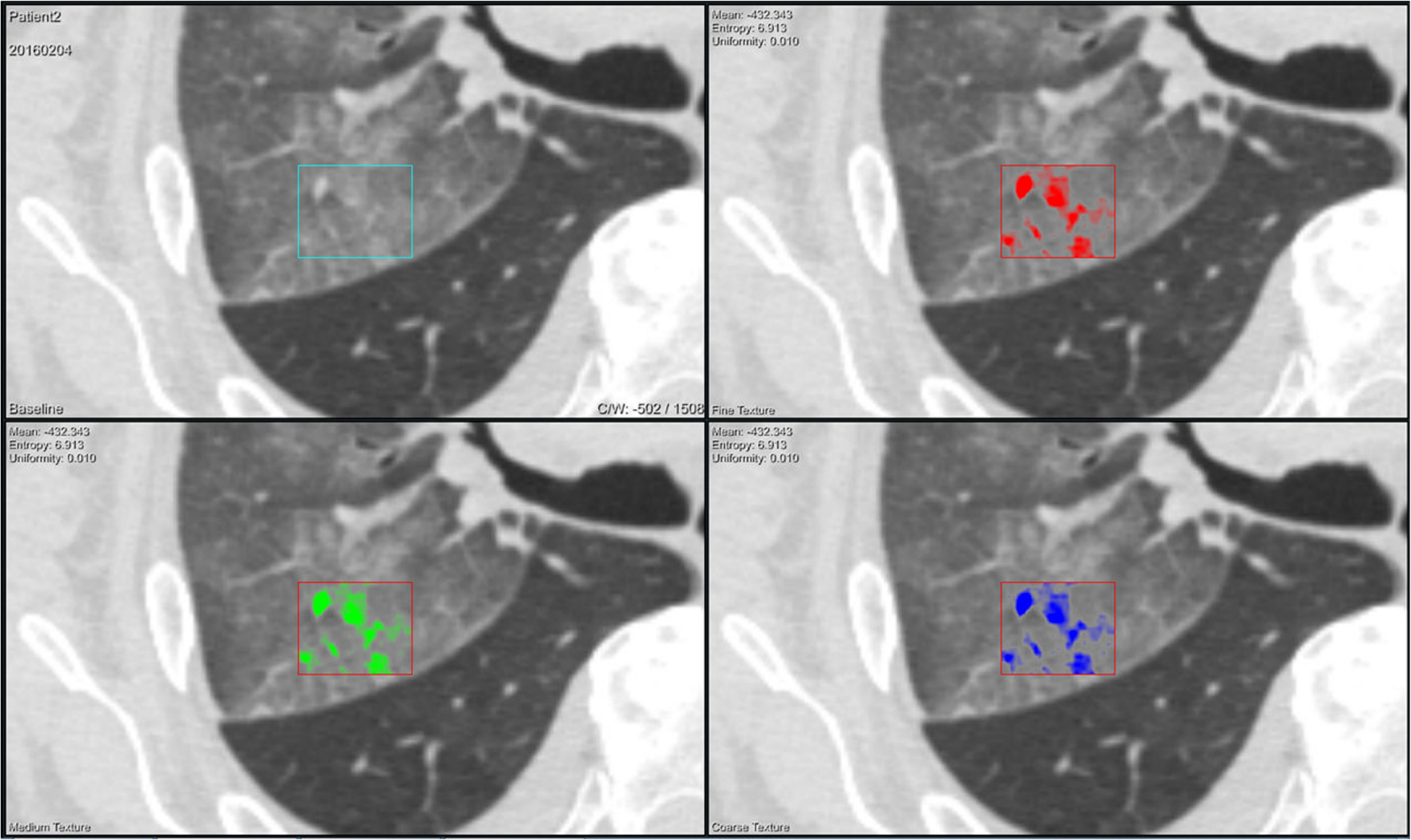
78-year-old male patient with lung hemorrhage following overdose of oral dabigatran anticoagulation therapy. Computed tomography texture analysis (CTTA) was applied on the detected haemorrhage in the right upper lobe These color-coded CTTA map display the mean intensity of the haemorrhage documented by a fine (*red*), medium (*green*), and course (*blue*) filter overlaying morphologic CT-image data
